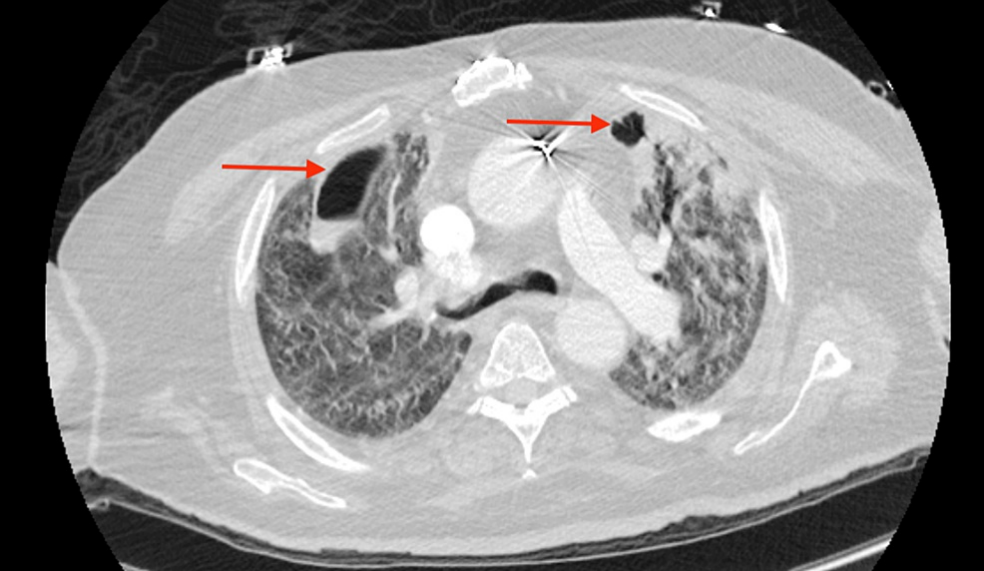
COVID-19 pneumonia complicated by superimposed cavitary bacterial pneumonia CT chest of a patient with COVID-19 pneumonia complicated by superimposed cavitary bacterial pneumonia. Axial image showing bilateral ground-glass opacities (GGOs) and multiple thick-walled cavitations involving the right middle and lower lobes.
